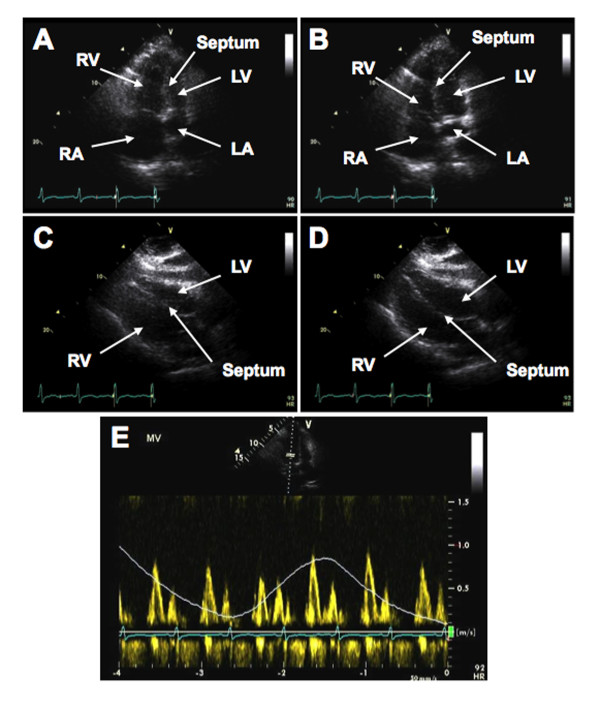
Transthoracic echocardiogram (TTE) showing abnormal ventricular and interventricular movement and mitral flow: TTE showing (a,b) marked interventricular movement; (c,d) restrictive movement of lateral ventricular walls with septal bounce; (e) tricuspid and mitral flow with inspiration and expiration. RA, LA, RV and LV correspond to the right and left atria and ventricles, respectively.
